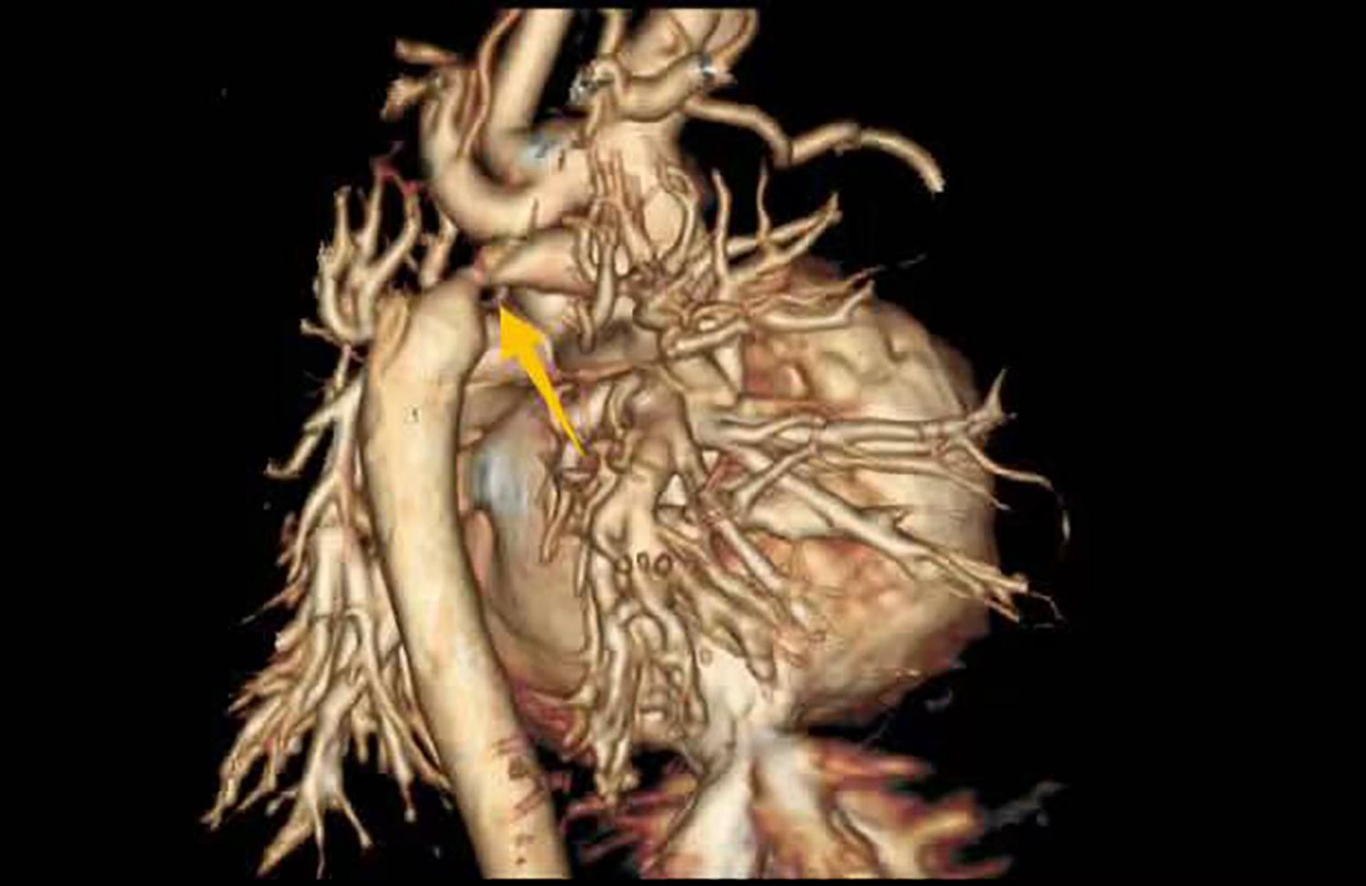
CT 3D reconstruction: persistent fifth aortic arch associated with stenosis (indicated by arrows) and an interrupted fourth aortic arch.
